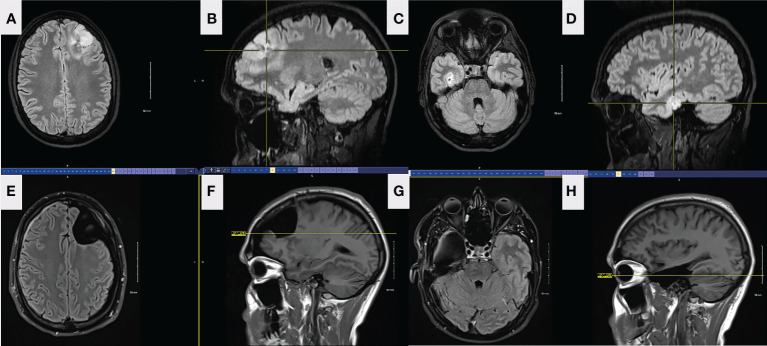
FLAIR axial (A) and sagittal (B) preop MRI of the left frontal lesion in patient 2. FLAIR axial (C) and sagittal (D) preop MRI of the right temporal lesion in patient 2. Axial (E) and sagittal (F) FLAIR MRI from left frontal tumor resection in patient 2, 5 months postoperatively. The resection was taken around all margins of the FLAIR lesion until functional borders were found, including into the corpus callosum across the midline. FLAIR MRI axial (G) and sagittal (H) from right temporal tumor resection in patient 2, 4 months postoperatively. The resection was taken beyond the margins of the FLAIR lesion.
